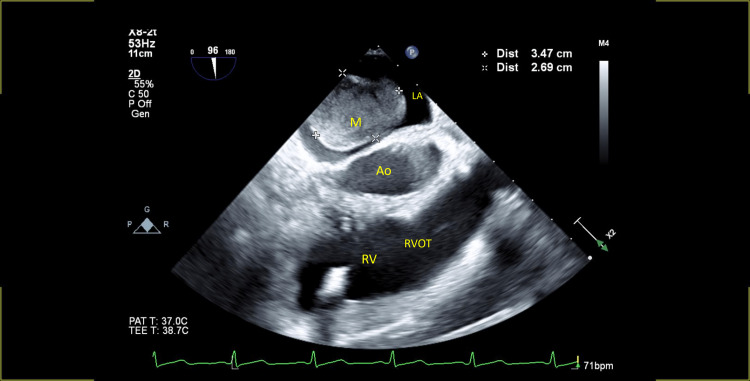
Transesophageal echocardiogram, mid-esophageal right ventricular inflow and outflow view showing an oval well-defined mass in the left atrium arising from the interatrial septum Ao - aortic root in short axis, LA - left atrium, M - mass, RV - right ventricle, RVOT - right ventricular outflow tract
